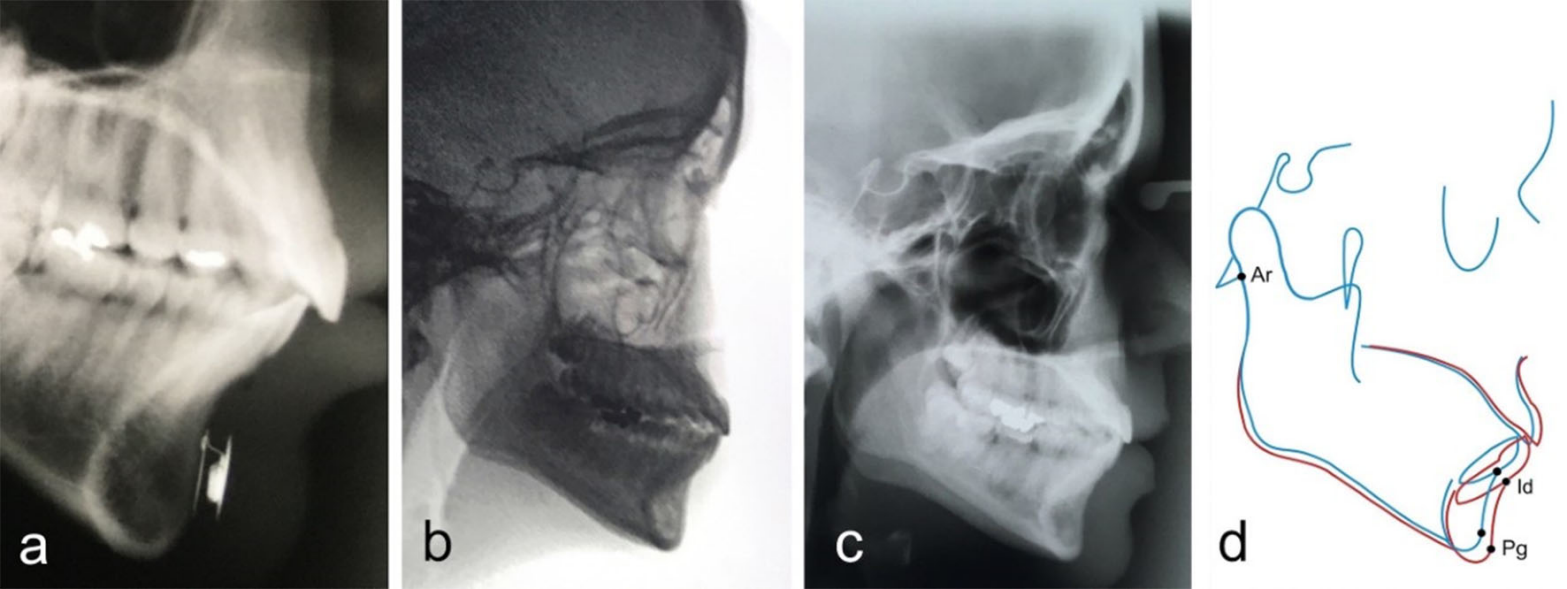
a Postoperative X-ray showing the Modulator properly located over the chin bone. The active plate is in contact with the chin bone. b Example of a preoperative cephalometric radiograph. c Long-term postoperative cephalometric radiograph of the same patient, taken 6 months after the removal of the Modulator. d Comparison between preoperative (*blue color*) and long-term postoperative (*red color*) traced cephalometric radiographs of this patient, showing a 7.60mm increase of mandible length and an approximately 3mm increase of the length of the vertical curve between pogonion and infradentale. Ar, articulare (junction between the inferior surface of the cranial base and the posterior border of the ascending ramus of the mandible); Pg, pogonion (most anterior point of mandibular symphysis); Id, infradentale.
